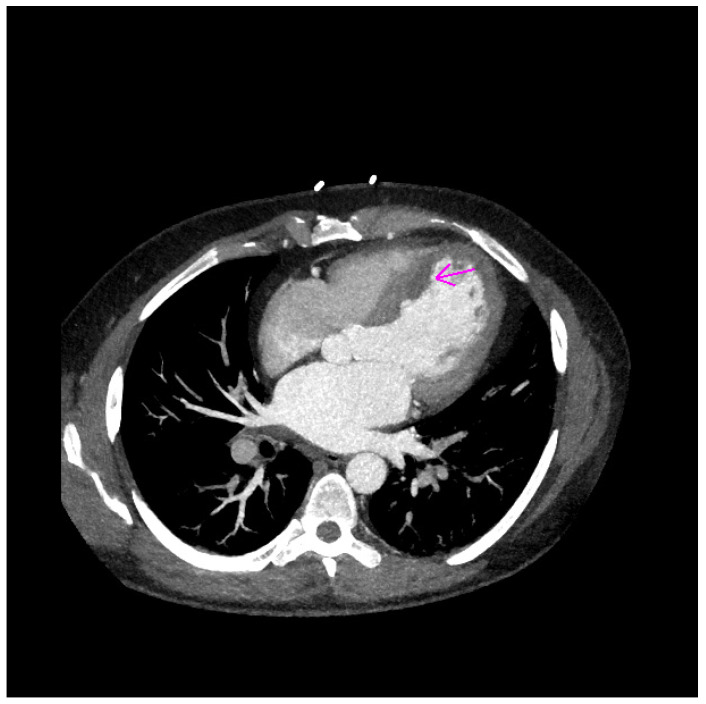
CT finding of second thrombus in the area of interventricular septum (purple arrow).
